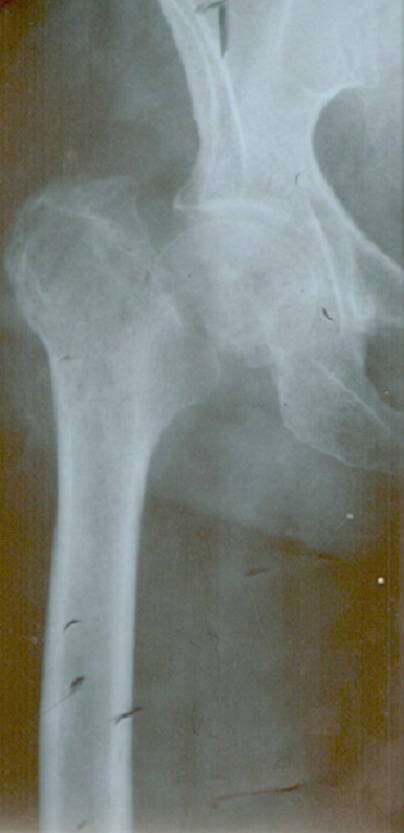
Radiographie de hanche de face montrant une pseudarthrose du col suite à une fracture negligee
